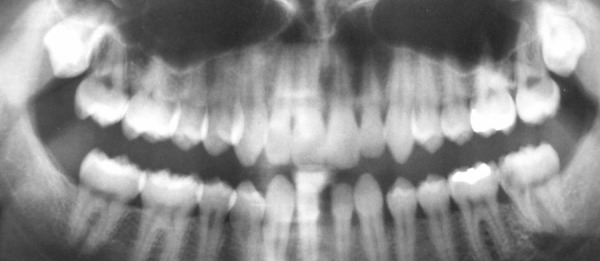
Panoramic radiography taken before emergency visit. Lower incisors and third molars are missing and upper third molars are impacted.
